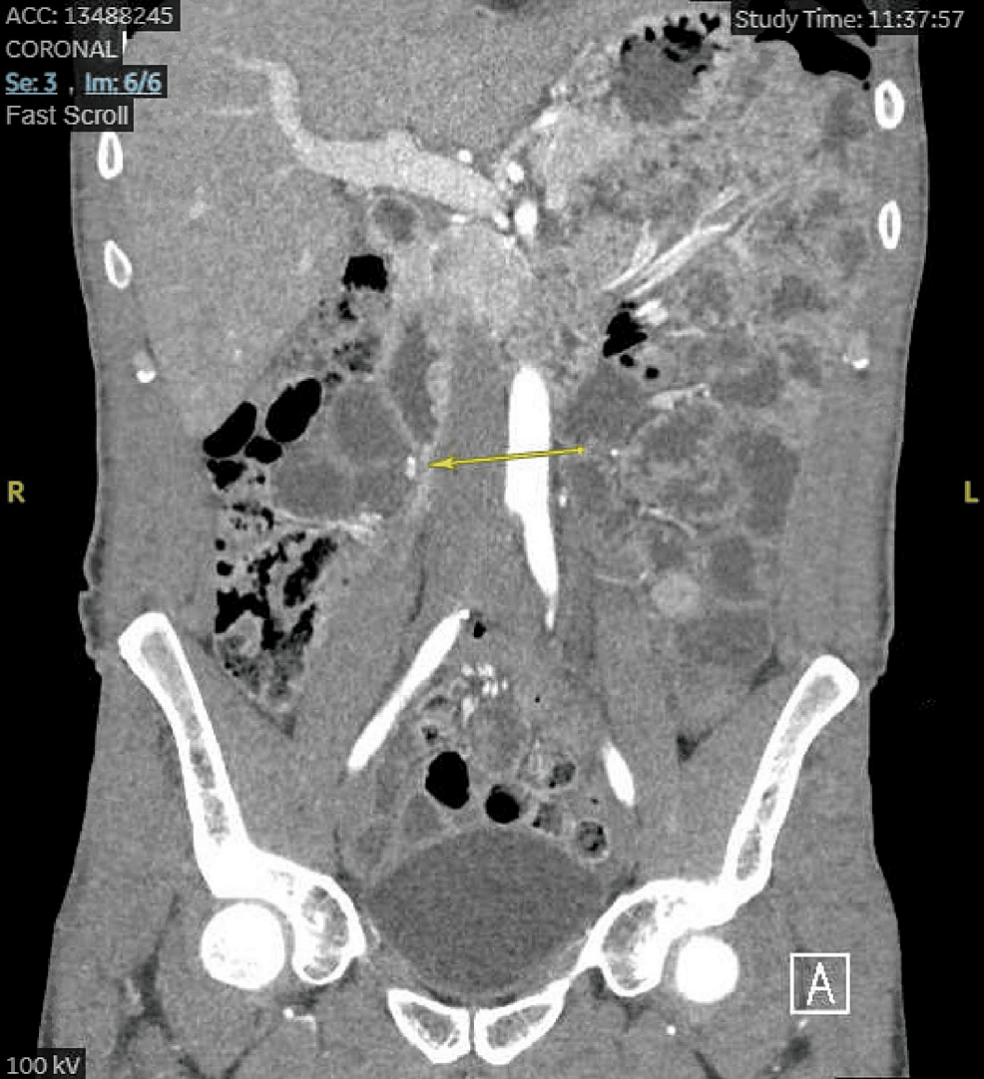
CT small bowel enterography (coronal view) showing soft tissue extending from the distal duodenum to the cecum
